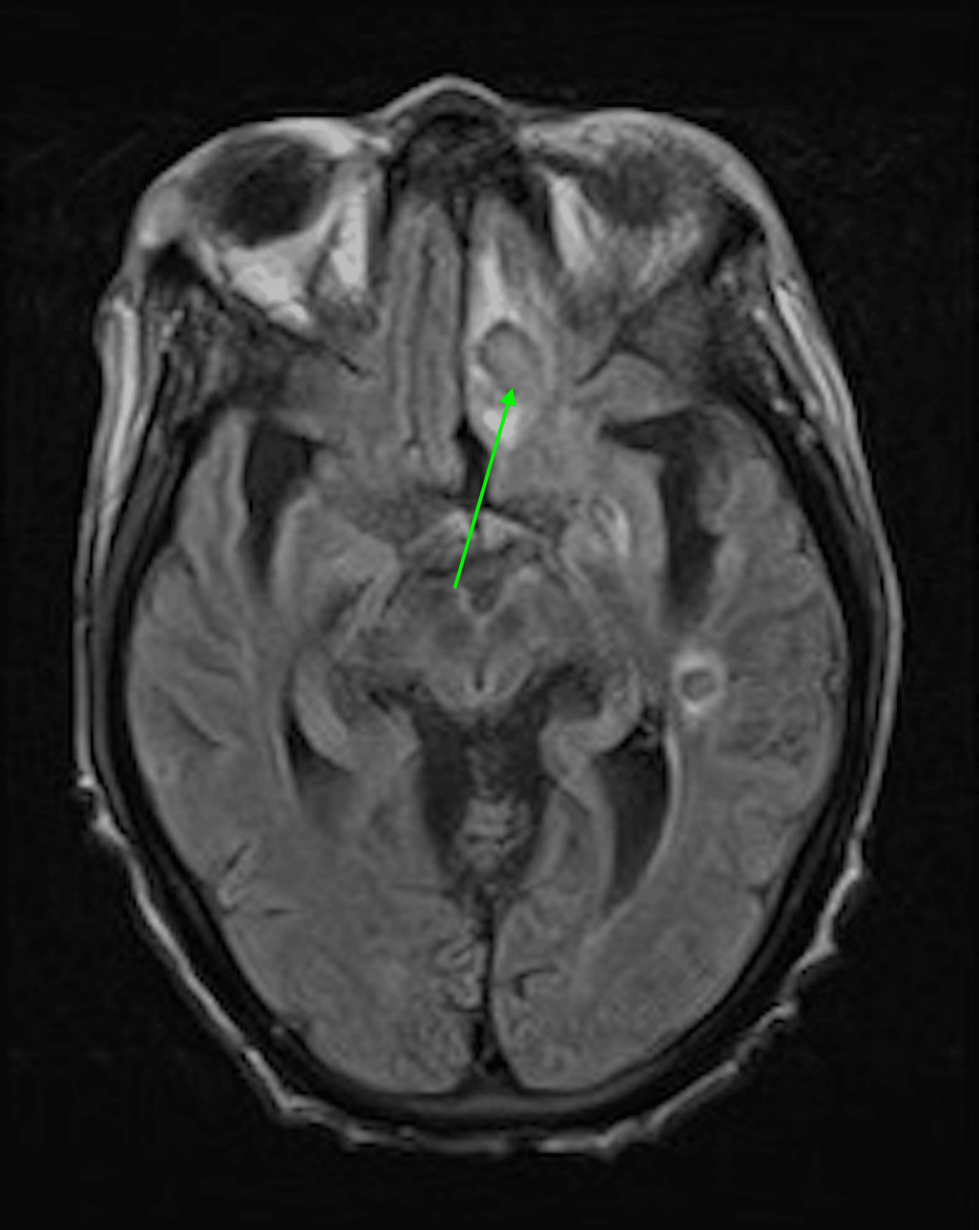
Follow-up MRI after the initiation of aggressive treatment.
